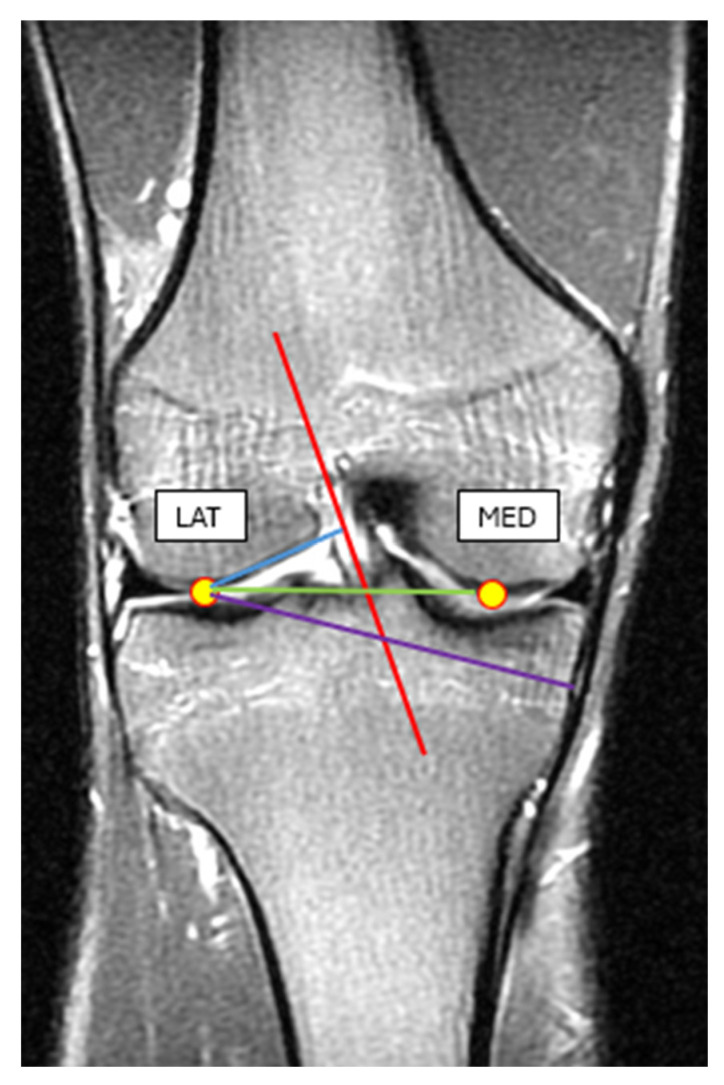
MRI image of the knee in an extended position. Blue line: the lateral condyle–ACL distance, purple line: lateral condyle–MCL distance, green line: lateral condyle–medial condyle distance.
